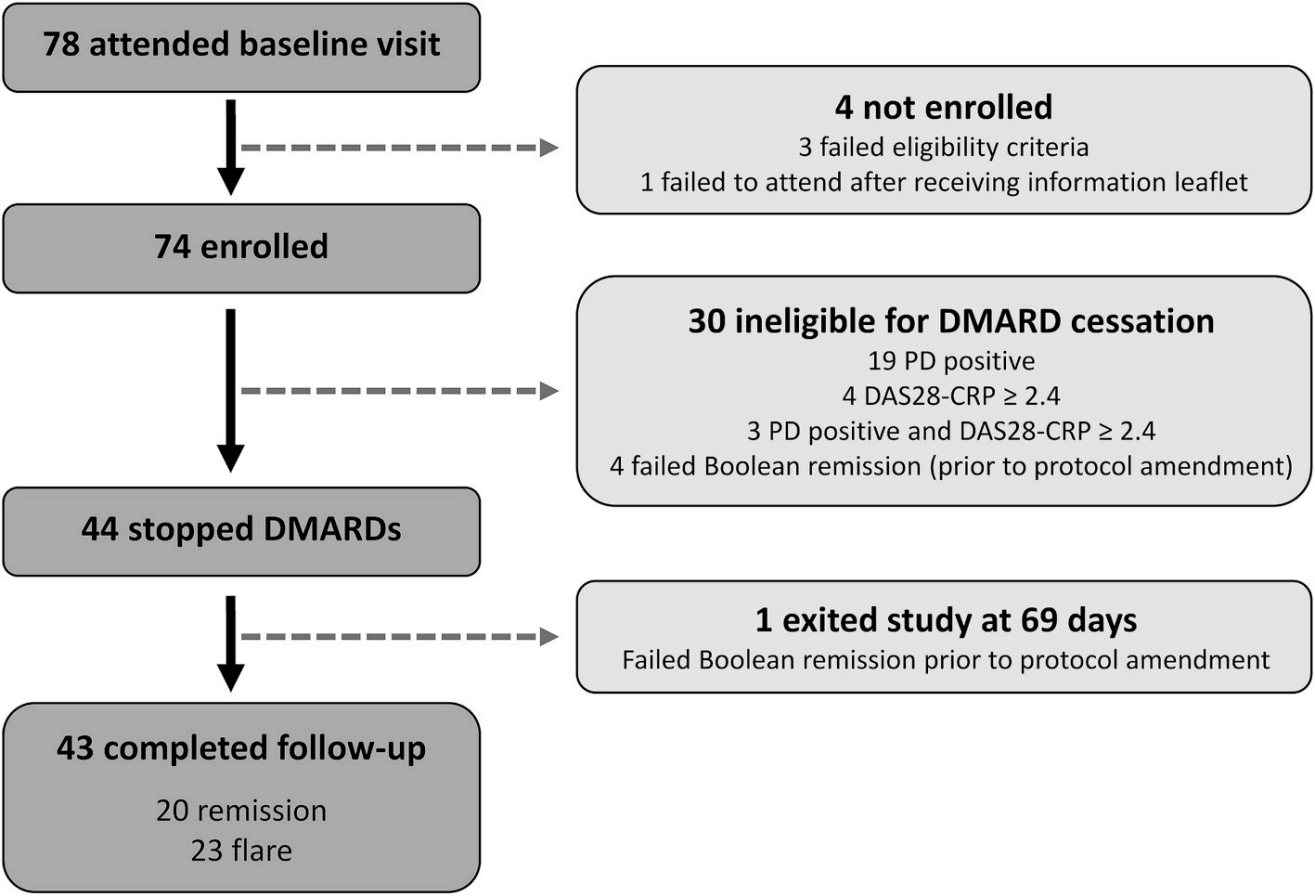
Study design and recruitment. 78 patients attended a baseline visit, of whom 44 stopped disease-modifying anti-rheumatic drug (DMARD) therapy. Patients then attended routine study visits at 1, 3 and 6 months following DMARD cessation, with additional unscheduled visits at the request of the patient in the event of suspected arthritis flare. Flare was confirmed if disease activity score in 28 joints with CRP (DAS28-CRP) ≥ 2.4, at which point the patient exited the study to restart DMARD therapy via their referring rheumatology team. Patients who maintained drug-free remission at 6 months remained without DMARDs and exited the study. PD: power Doppler.
